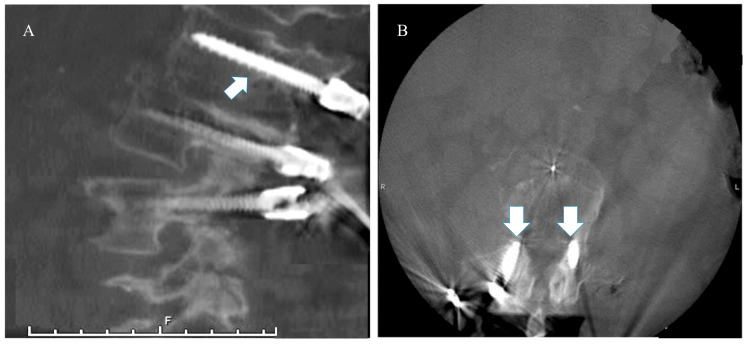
The intraoperative CT scan shows the conversion of the cortical screw to the pedicle screw (white arrow) in the sagittal (A) and axial (B) frame. The new pedicle screw follows a medial-to-lateral path and is parallel to the superior endplate.
